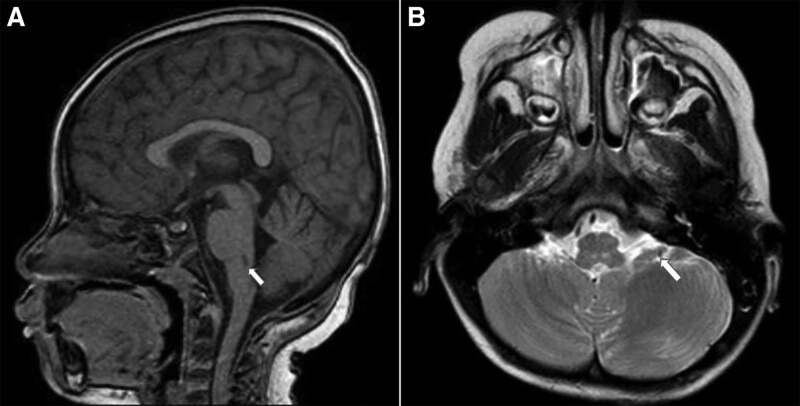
There was an improvement in MRI findings, with downsized nodular lesions in the posterior medulla oblongata bilaterally, as indicated by white arrows in the sagittal plane (A) and axial views (B).
